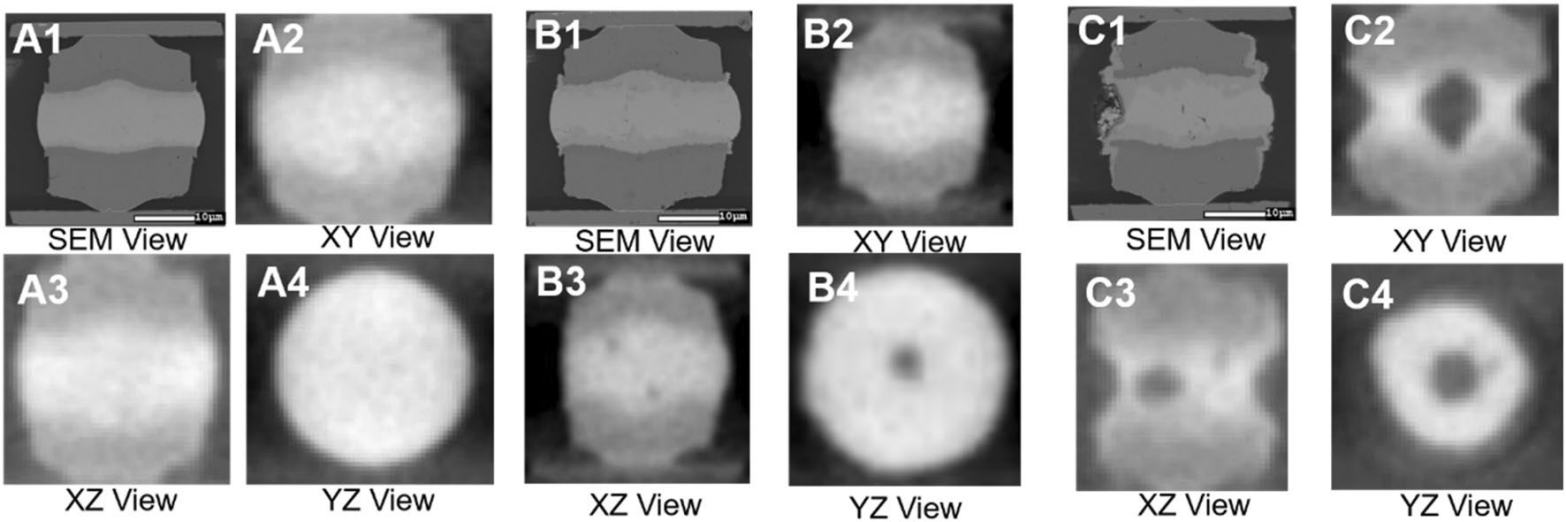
SEM images and CT images in the XY, XZ, and YZ planes of a selected microbump at (A) the initial state, the state of the resistance increased by (B) 10% and (C) 20%.
